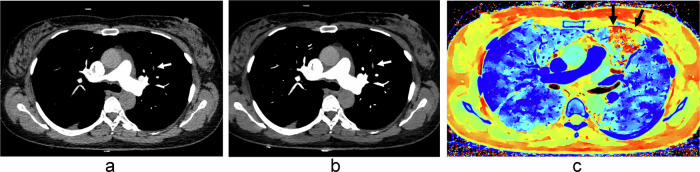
60-year-old woman with suspected acute pulmonary embolism. CT pulmonary angiography with contrast administration using protocol C shows a subsegmental pulmonary embolism in a branch of the artery for the anterior segment of the left upper lobe (white arrows in a and b). One reader missed the clot in conventional images (a) and 45 keV virtual monoenergetic image (b) but detected the perfusion defect in the Z-effective map (black arrows in c). The other reader diagnosed pulmonary embolism in all three images
